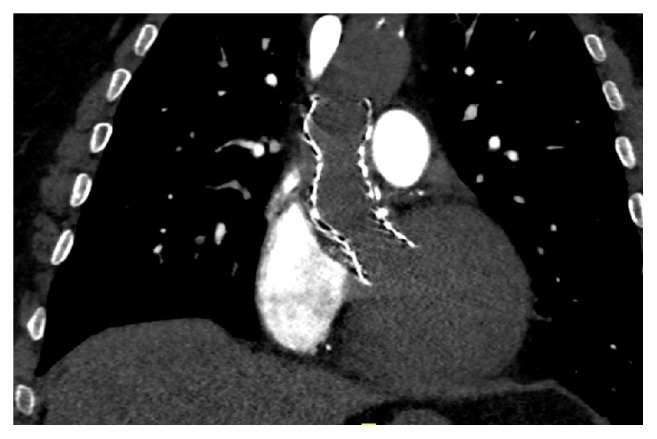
Computed tomography showing positions of TAVI in TAVI.
